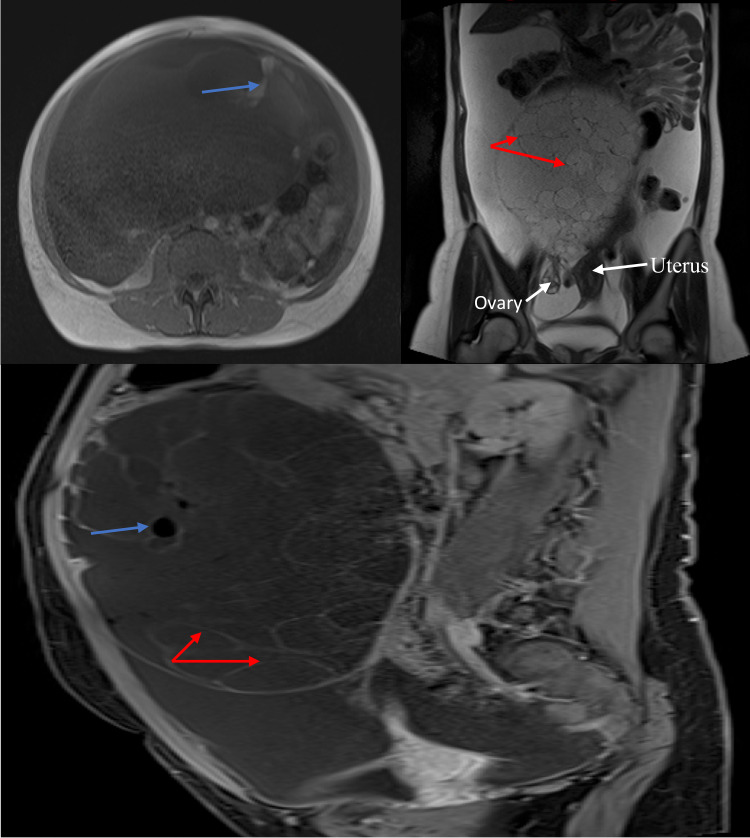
Axial, coronal, and sagittal MRI of the abdomen and pelvis showing large left ovarian multilocular cystic mass, see the enhancing septations (red arrows). The macroscopic fat shows bright signal in the non-fat saturated T2 FSE with corresponding drop in signal in the T1 fat suppressed post-contrast image (blue arrow). FSE, fast spin echo
